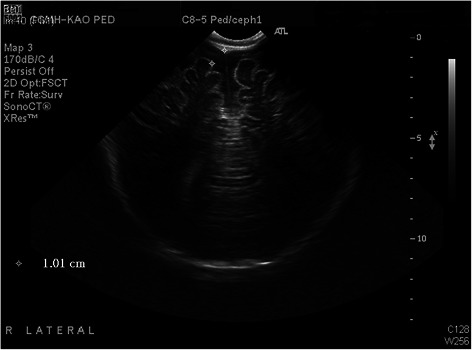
Brain ultrasound. Initial brain ultrasound on admission with moderate subdural fluid collection(1.01 cm)
